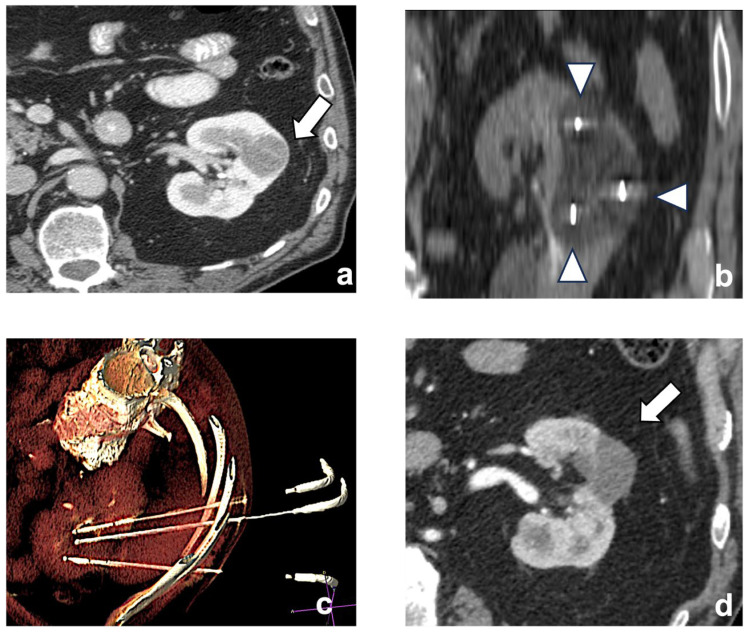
A left chromophobe RCC (arrow), hypovascular compared to normal renal cortex (a). Three cryoablation probes (arrowheads) are placed in the tumor, creating an ice-ball ((b) coronal plane). A volume-rendering reconstruction represents the probes’ access and positioning in the renal mass (c). Post-contrast CT shows the positive outcome of the ablation (arrow (d)).
